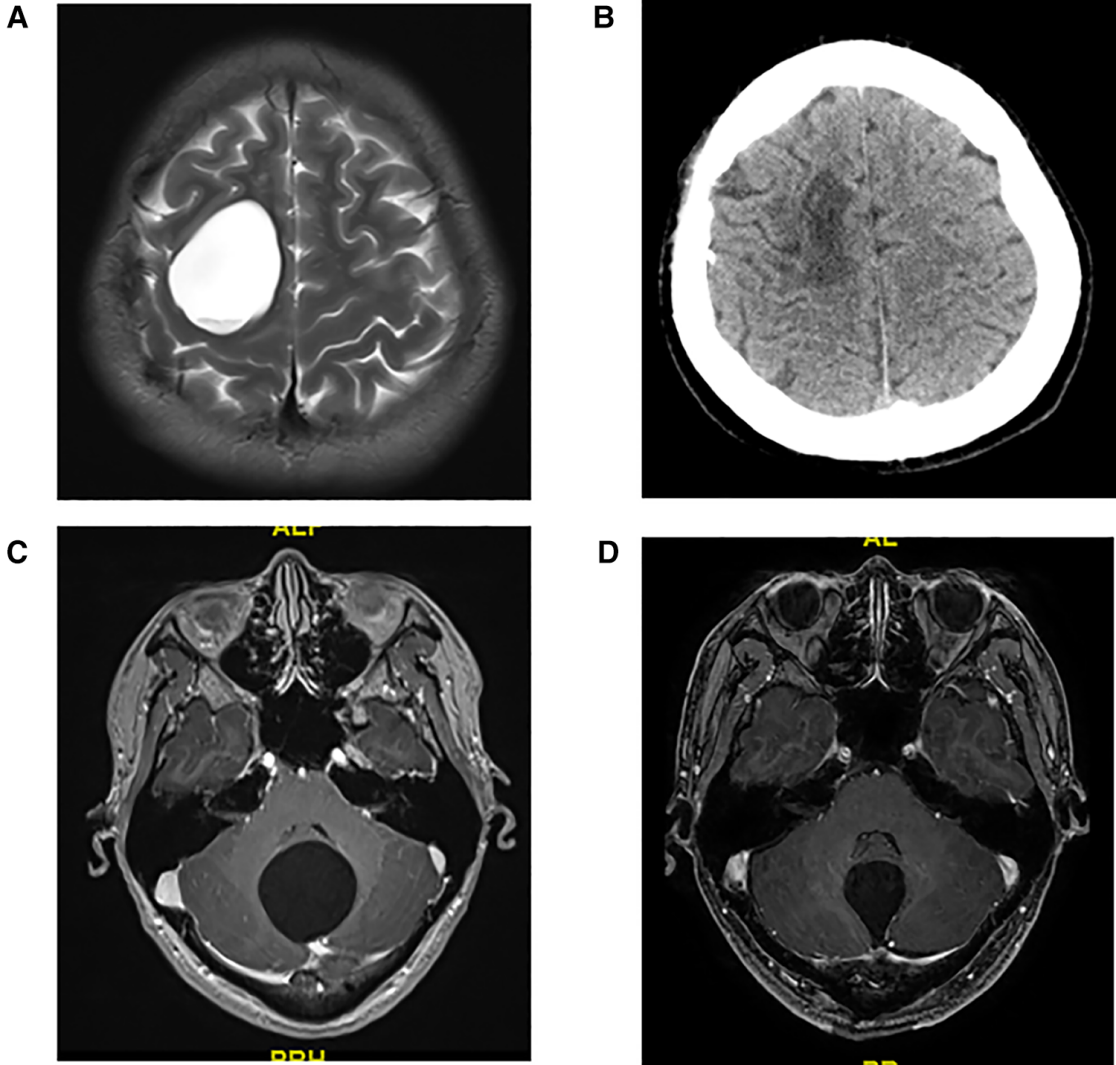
(A) Preoperative T2 MRI image of No. 7 patient. (B) CT image of No. 7 patient at 1 month after neuroendoscopic surgery. (C) Preoperative T1 MRI image of No. 11 patient. (D) T1 MRI image of No. 11 patient at 1 week after craniotomy.
